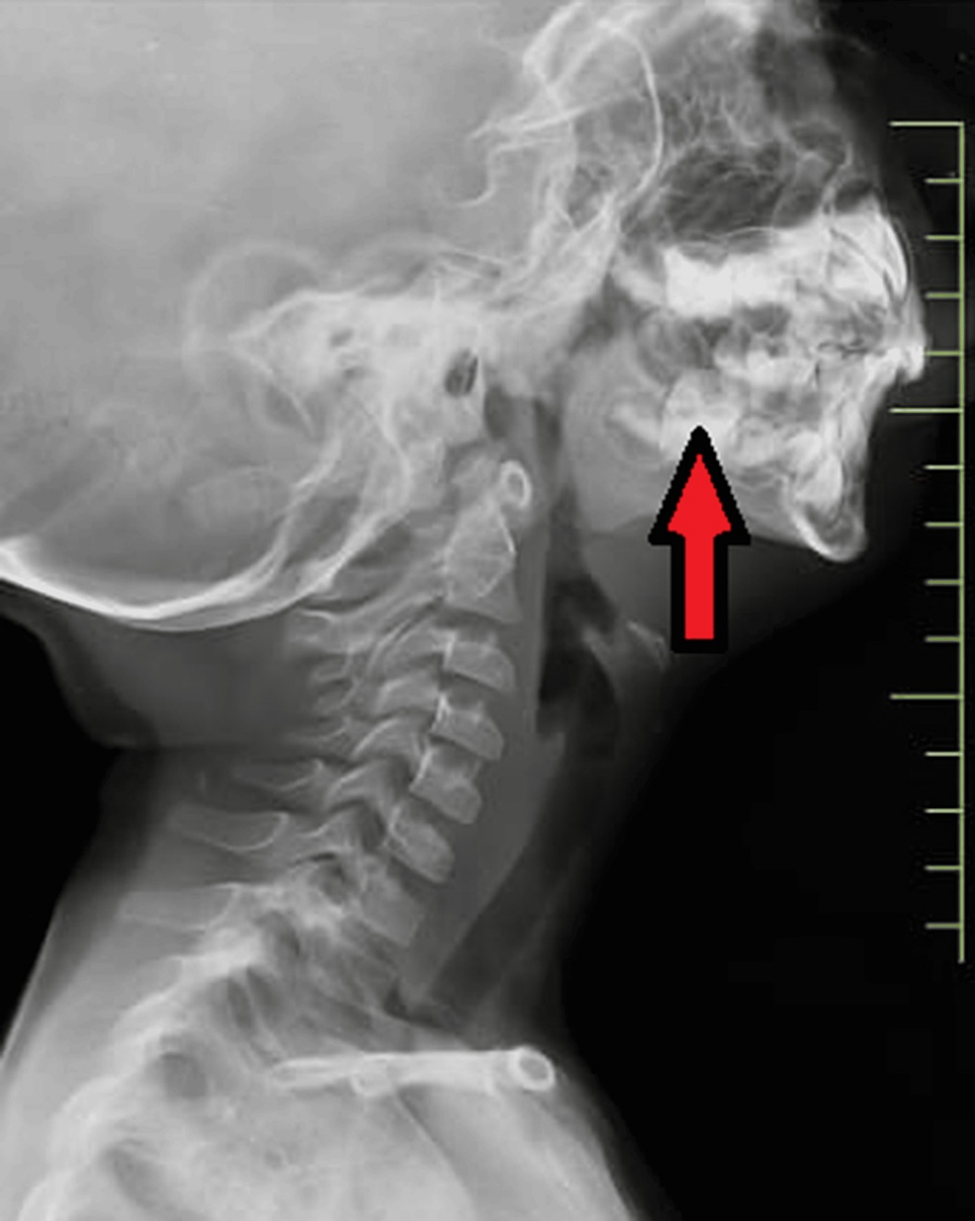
X-ray of the nasopharynx showing grade 3 adenoid hypertrophy.
